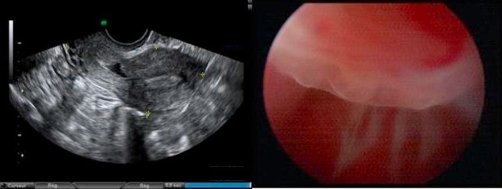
Uterine defect within the Caesarean section scar 4 months after treatment: (Left) endovaginal ultrasonography; (Right) hysteroscopy.
